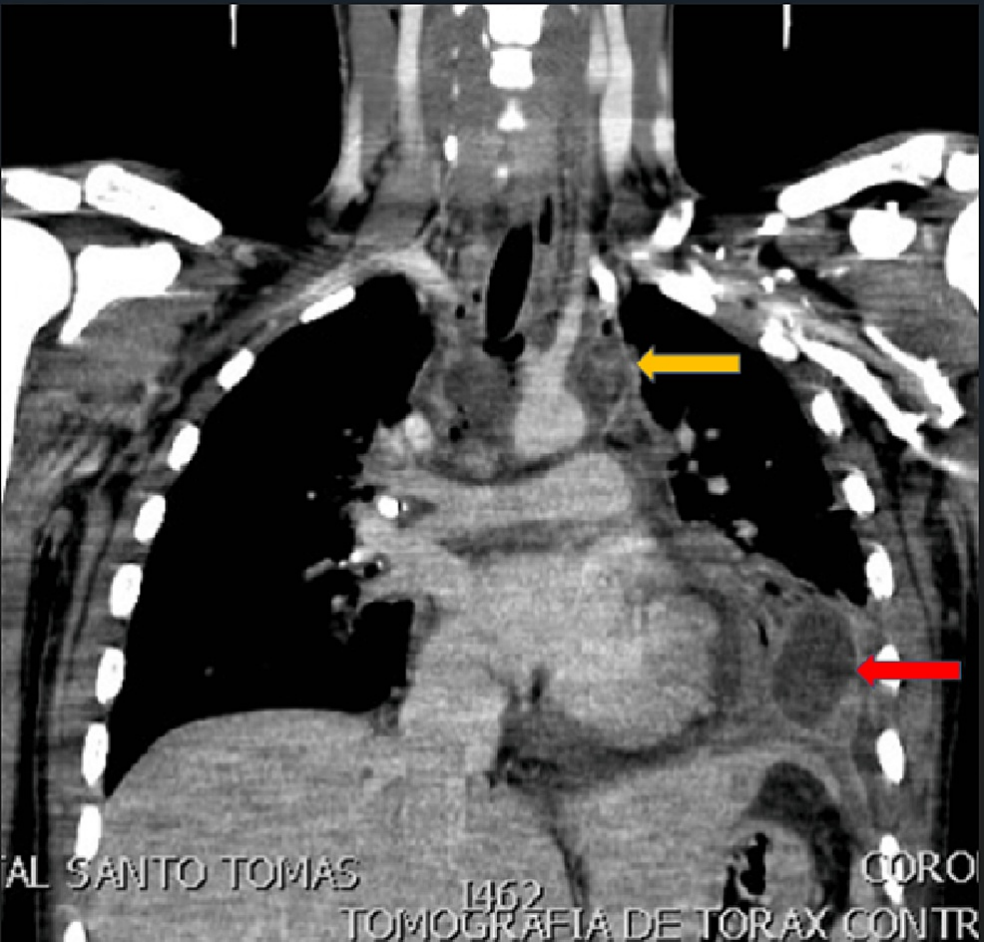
CT scan of the chest w/contrast, coronal view. Note mediastinal abscess (yellow arrow) and loculated empyema (red arrow).
